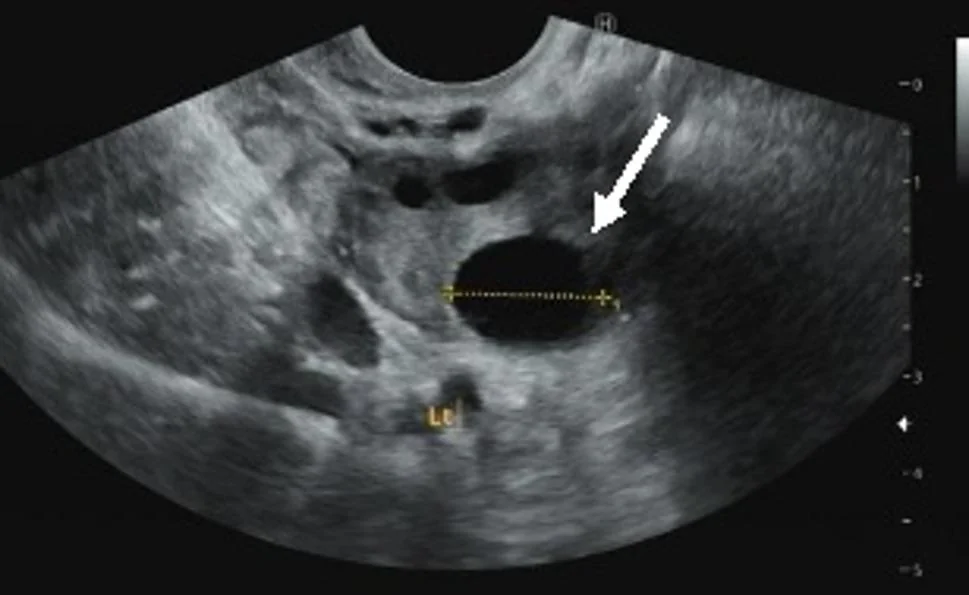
Transvaginal ultrasound performed one month after surgery shows follicular development in the left ovary, the affected side (arrow)
